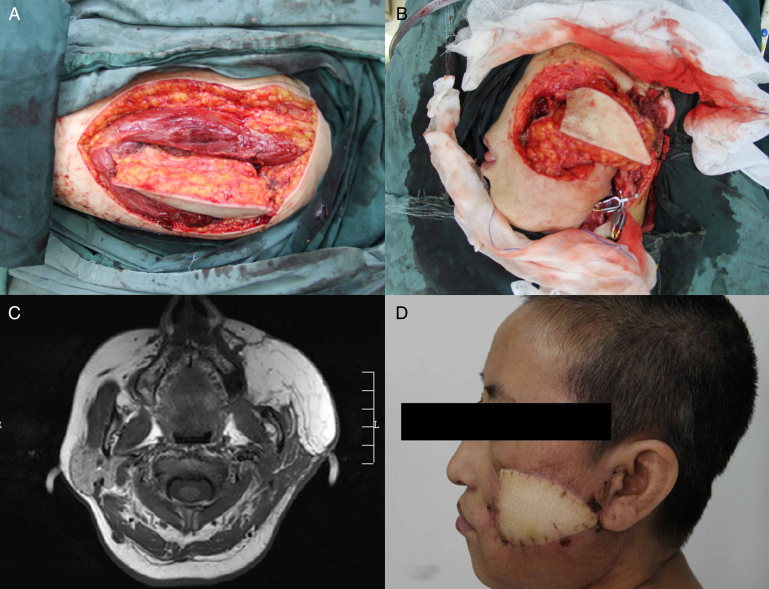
(A) The ALT flap was harvested from the left thigh; (B) the through-and-through defect over left cheek after excision of buccal squamous cell carcinoma; (C) postoperative axial MRI showing the ALT flap; (D) postoperative result 4 months after operation.
